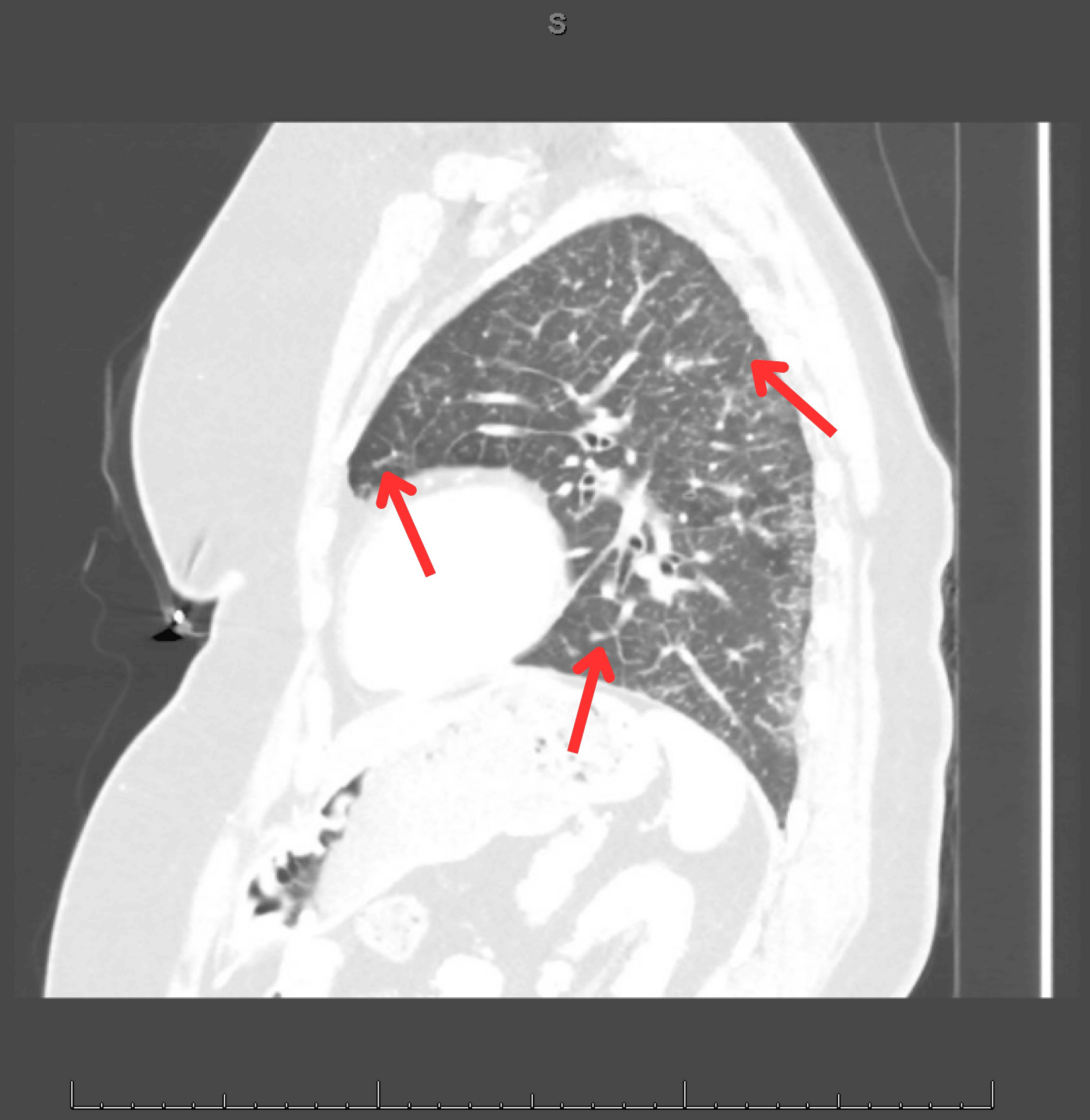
Sagittal reconstruction of the chest CT. The linear opacities and nodular thickening further support the tree-in-bud appearance. All red arrows indicate linear opacities due to small airway thickening and bronchiolar plugging.
